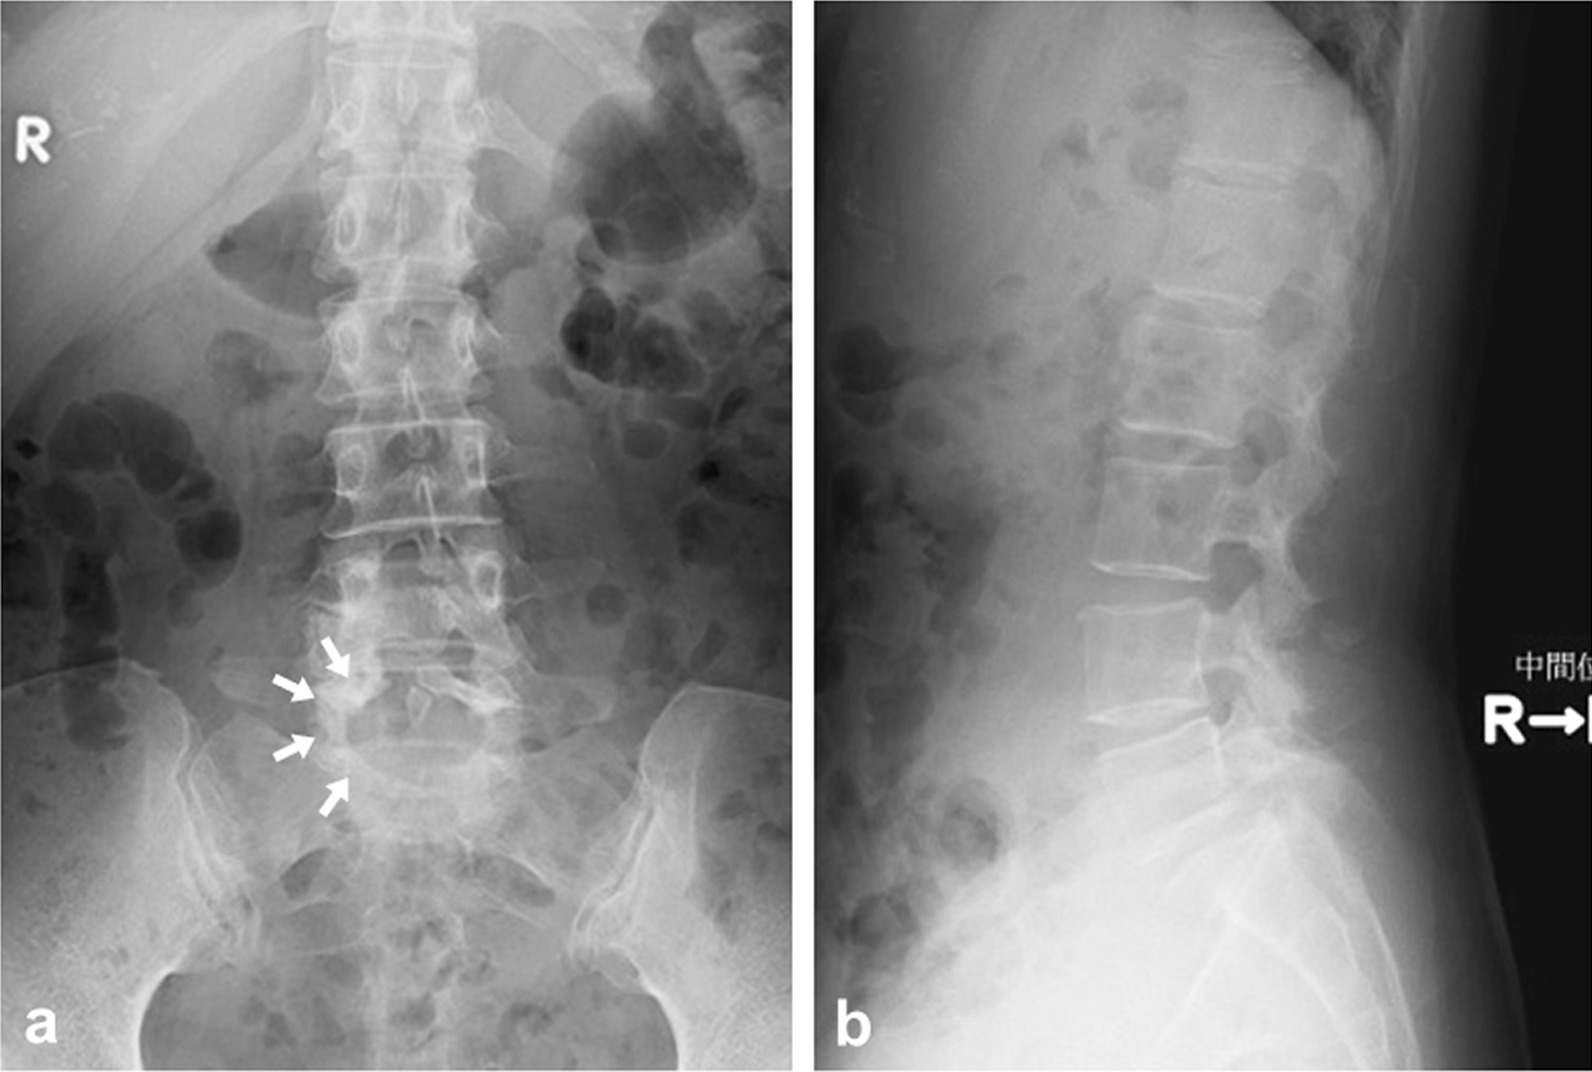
Plain radiographs at 6 months after surgery. Wide fenestration at the right L5/S1 interlaminar space was performed (white arrows). a a-p view. b lateral view
